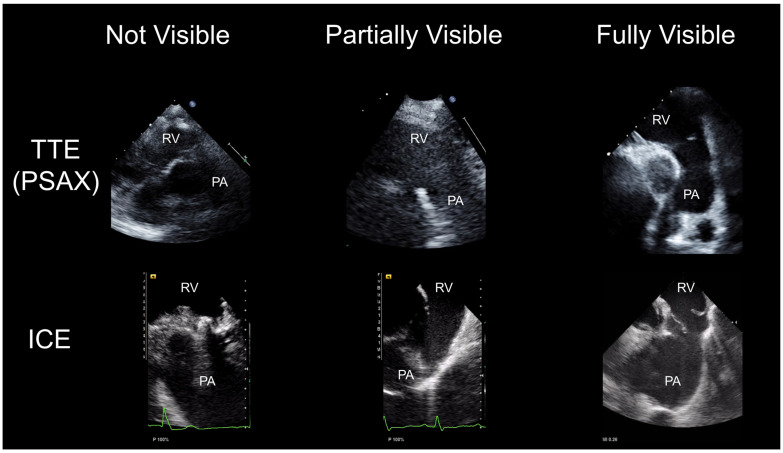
Echocardiographic assessment of PV using TTE and ICE. Representative imaging of the “not visible”, “partially visible” and “fully visible” classifications by TTE and ICE. The upper and lower images are not of the same patients. TTE, transthoracic echocardiography; ICE, intracardiac echocardiography; RV, right ventricle; PA, pulmonary artery; PSAX, parasternal short axis.
